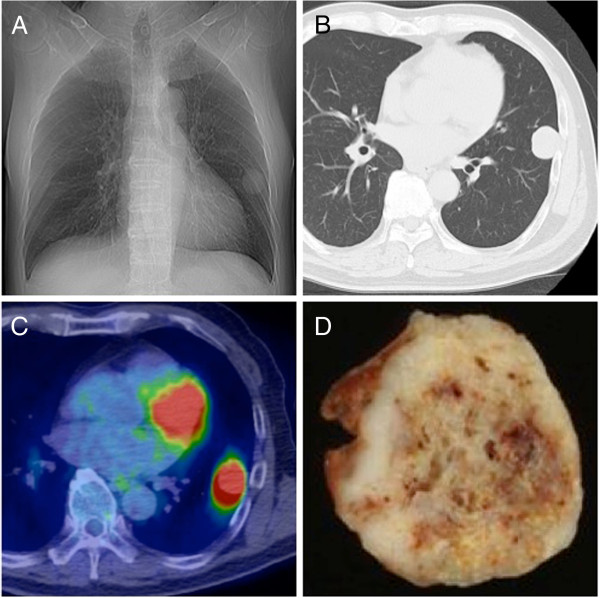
Imaging findings. (A) Plain chest radiograph revealed a mass in the left middle lung field. (B) Computed tomography (CT) revealed a left lung tumor (28 × 22 mm) with smooth margin. (C) Positron-emission tomography revealed fluorodeoxyglucose accumulation in the mass. (D) Macroscopic appearance of the resected tumor. The tumor was solid and white with a smooth margin.
